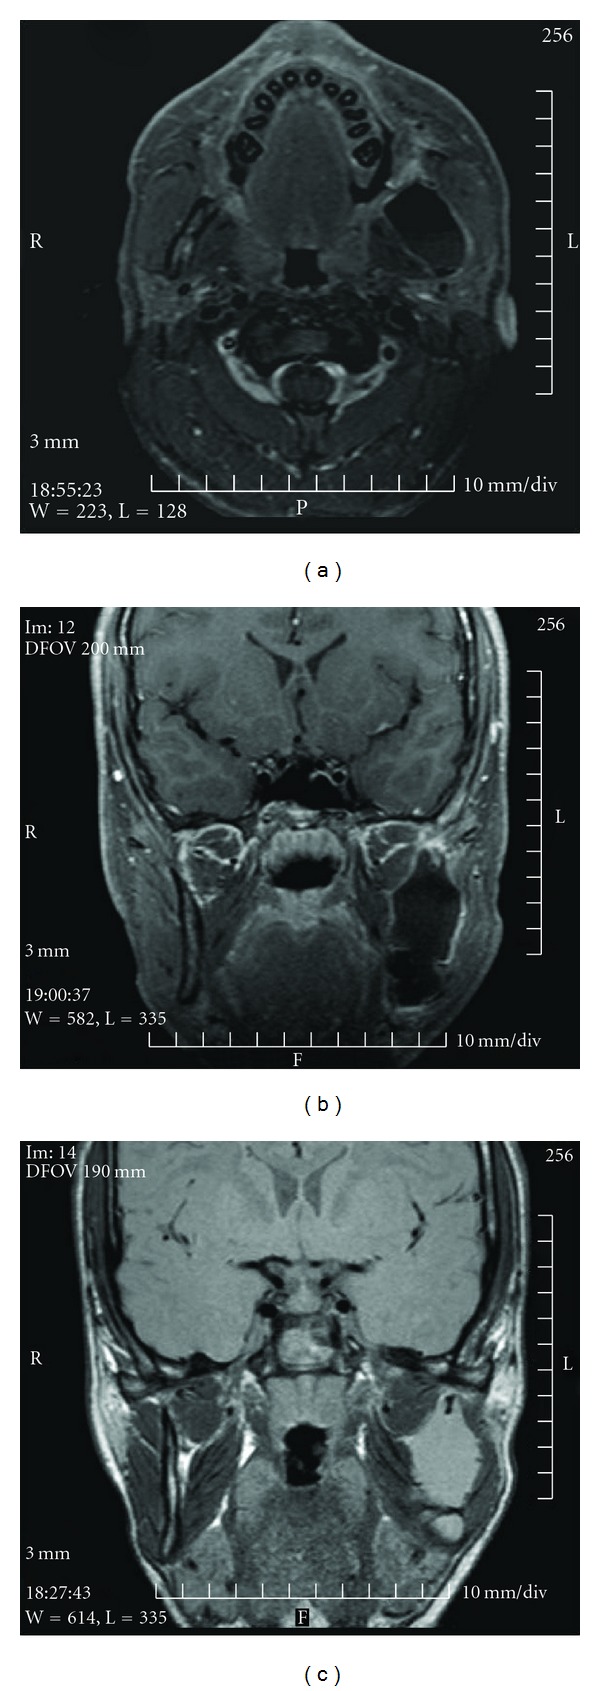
MR images demonstrate the low signal intensity on T1-weighted axial (a) and sagittal (b) images and the high signal intensity on T2-weighted sagittal images (c).
